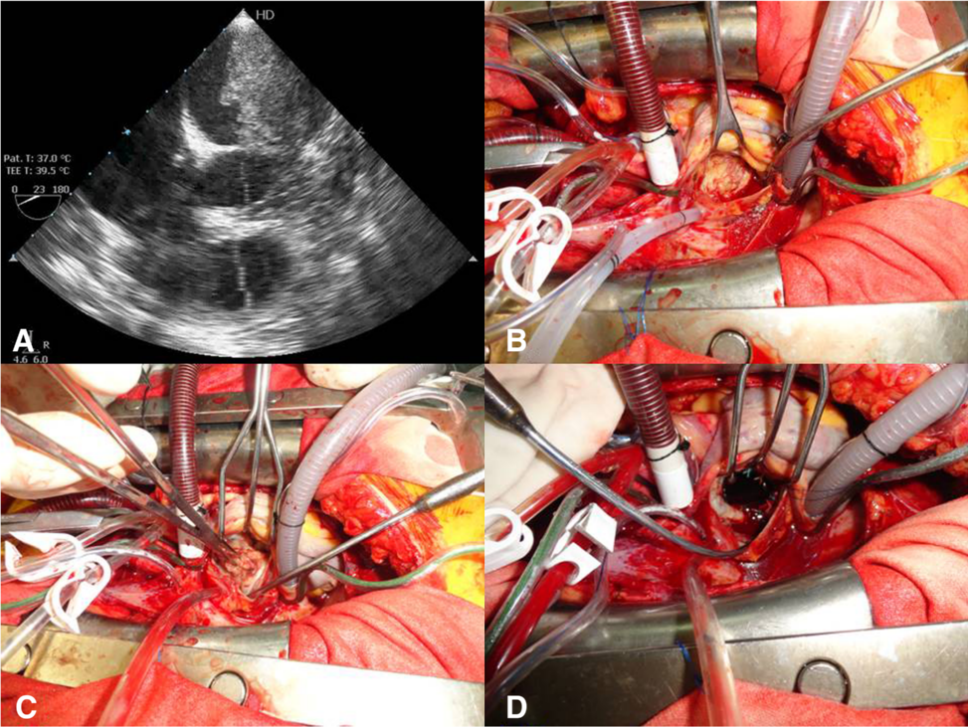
a Intraoperative transesophageal echocardiography. b Yellowish-white mass in the left atrium. c Removal of the mass from the endocardium. d Mitral valve replacement with a mechanical prosthesis
